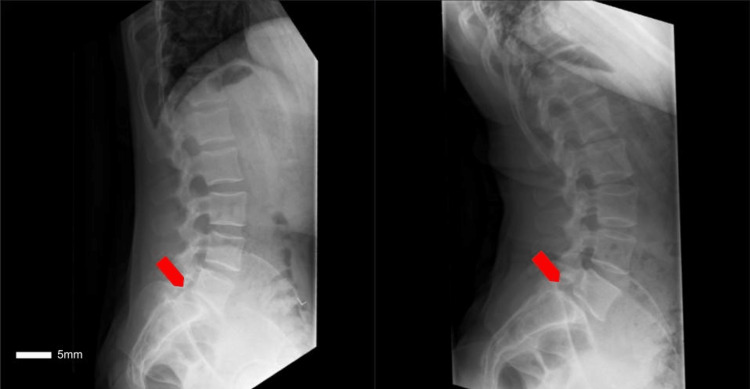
A flexion (left) and extension (right) lateral plain film radiograph of the lumbar spine demonstrating a mobile spondylolisthesis at L5-S1 (red arrows)
